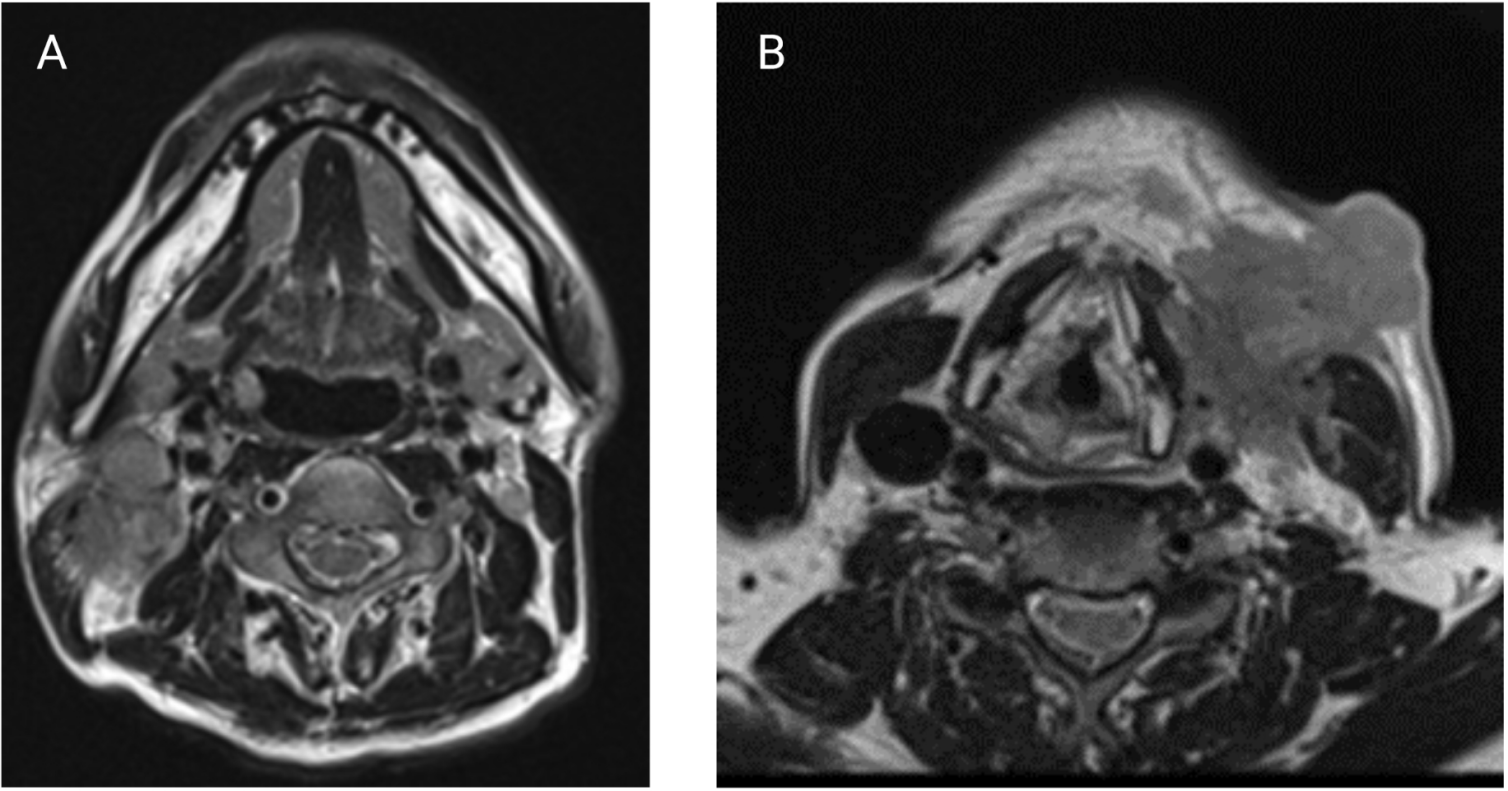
(A) Axial T2-weighted MRI showing a coalescent right level II nodal mass suspicious for iENE. (B) Axial T2-weighted MRI with a large L level III nodal mass with clear invasion of the sternocleidomastoid and extension into overlying subcutaneous fat and skin. (Images kindly provided by Dr. Eugene Yu).
